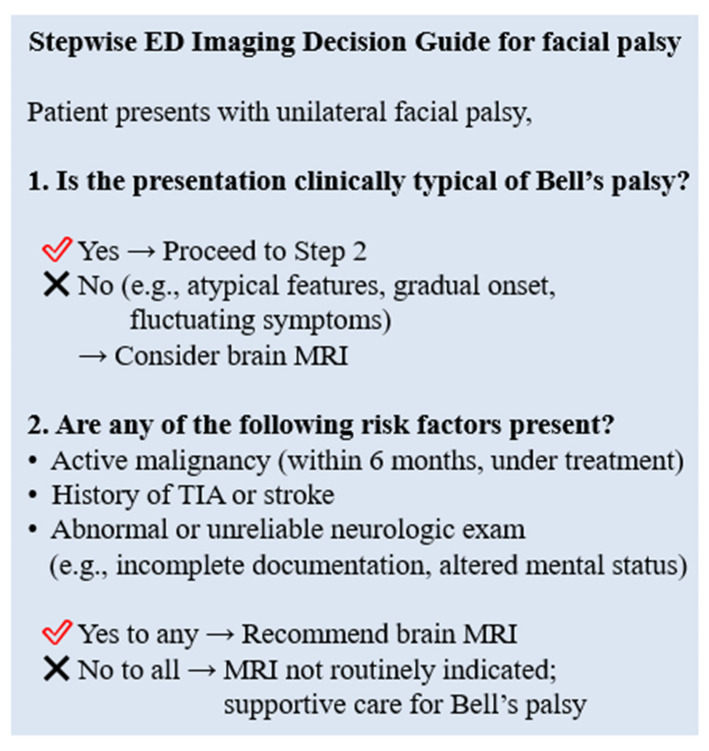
Stepwise emergency department imaging algorithm for unilateral facial palsy. MRI—magnetic resonance imaging; TIA—transient ischemic attack.
